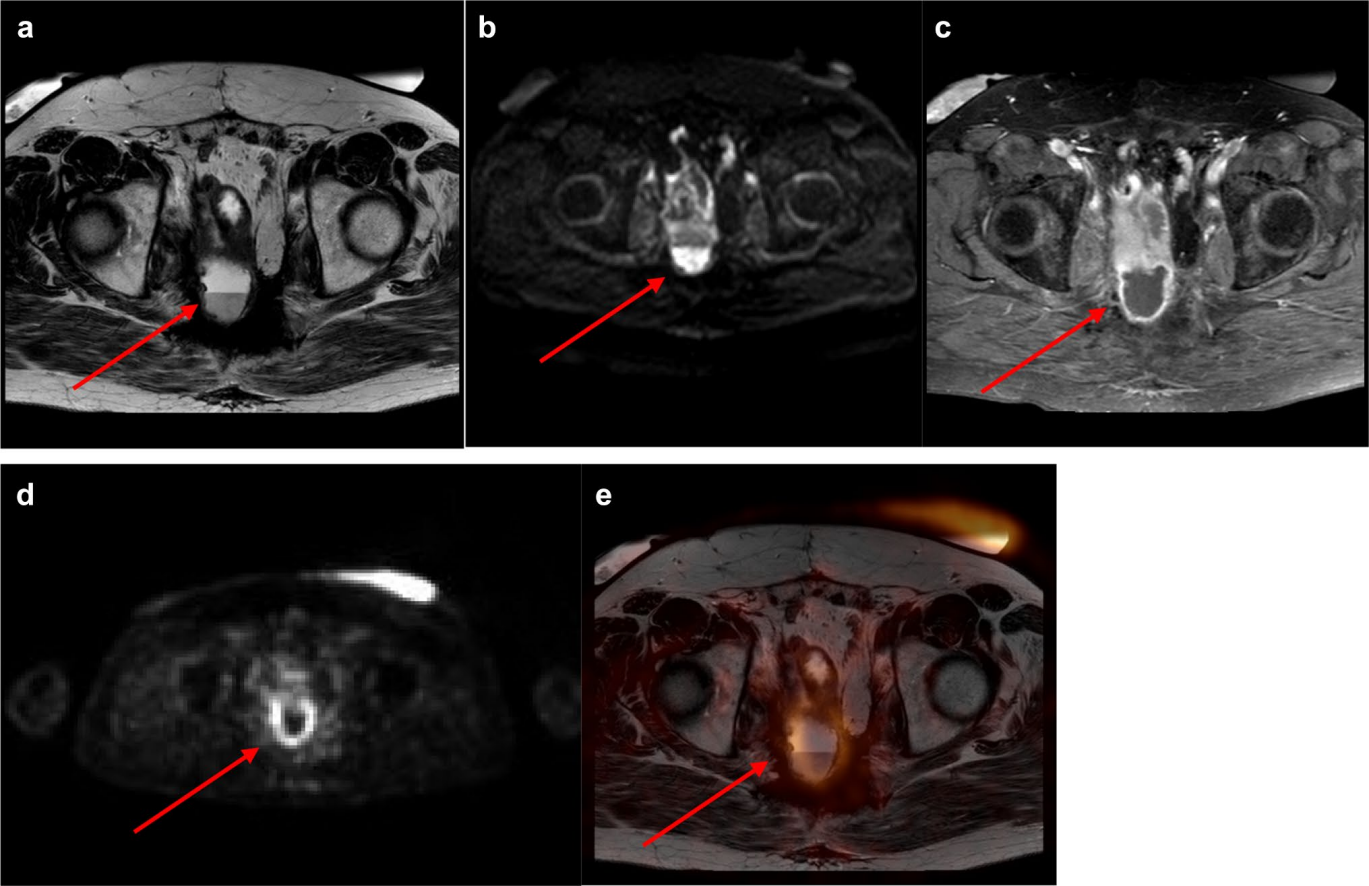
a–d Chronic inflammation with presacral fluid collection. a–c Axial T2-weighted/axial diffusion-weighted/axial T1-weighted contrast-enhanced MRI. MRI showing a presacral, partially T2-hyperintense lesion with a thick hypointense rim and partially restricted diffusion, fluid-level and contrast enhancement of the rim. MRI was scored as equivocal (score 2). d/e Corresponding PET and PET/MRI fusion images demonstrating slightly increased FDG-uptake presacrally. PET/MRI was scored as equivocal (score 2). Follow-up of more than 4 years excluded tumor recurrence
